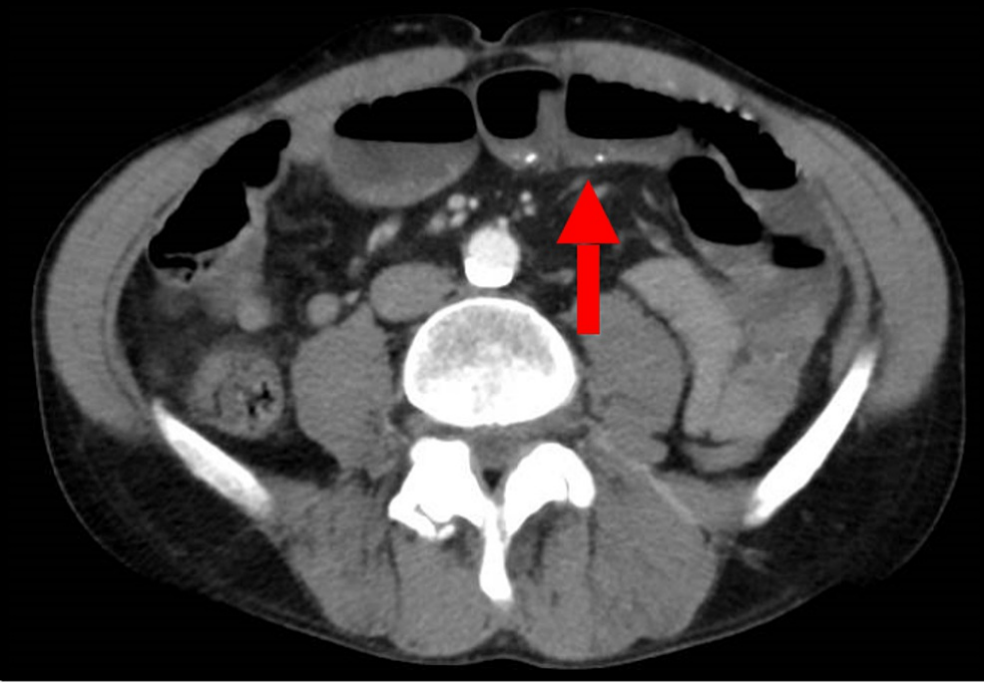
CT scan - transverse section (image 1) Arrow indicates air-fluid levels suggesting small bowel obstruction CT: computed tomography
